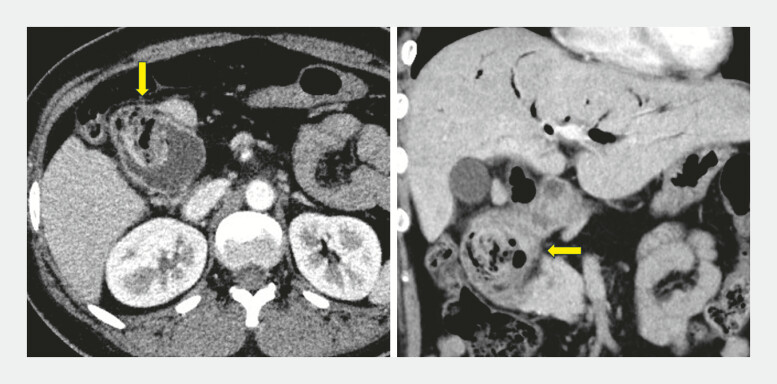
Arterial phase of contrast-enhanced computed tomography (CT) revealed that a large stone in the dilated bil duct partially protruded into the duodenum in the axial view (left). The coronal CT scan revealed pneumobilia in the intrahepatic bile ducts; a large stone partially protruded into the duodenum (right).
